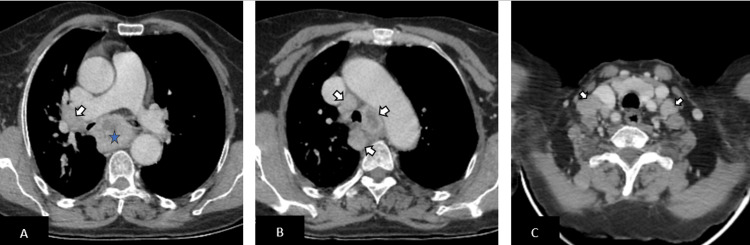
Baseline axial contrast-enhanced CT images (A, B, and C) revealed enlarged lymph nodes in the right hilar, pretracheal, paratracheal, and supraclavicular (arrows), consistent with multifocal mediastinal and cervical nodal involvement.
